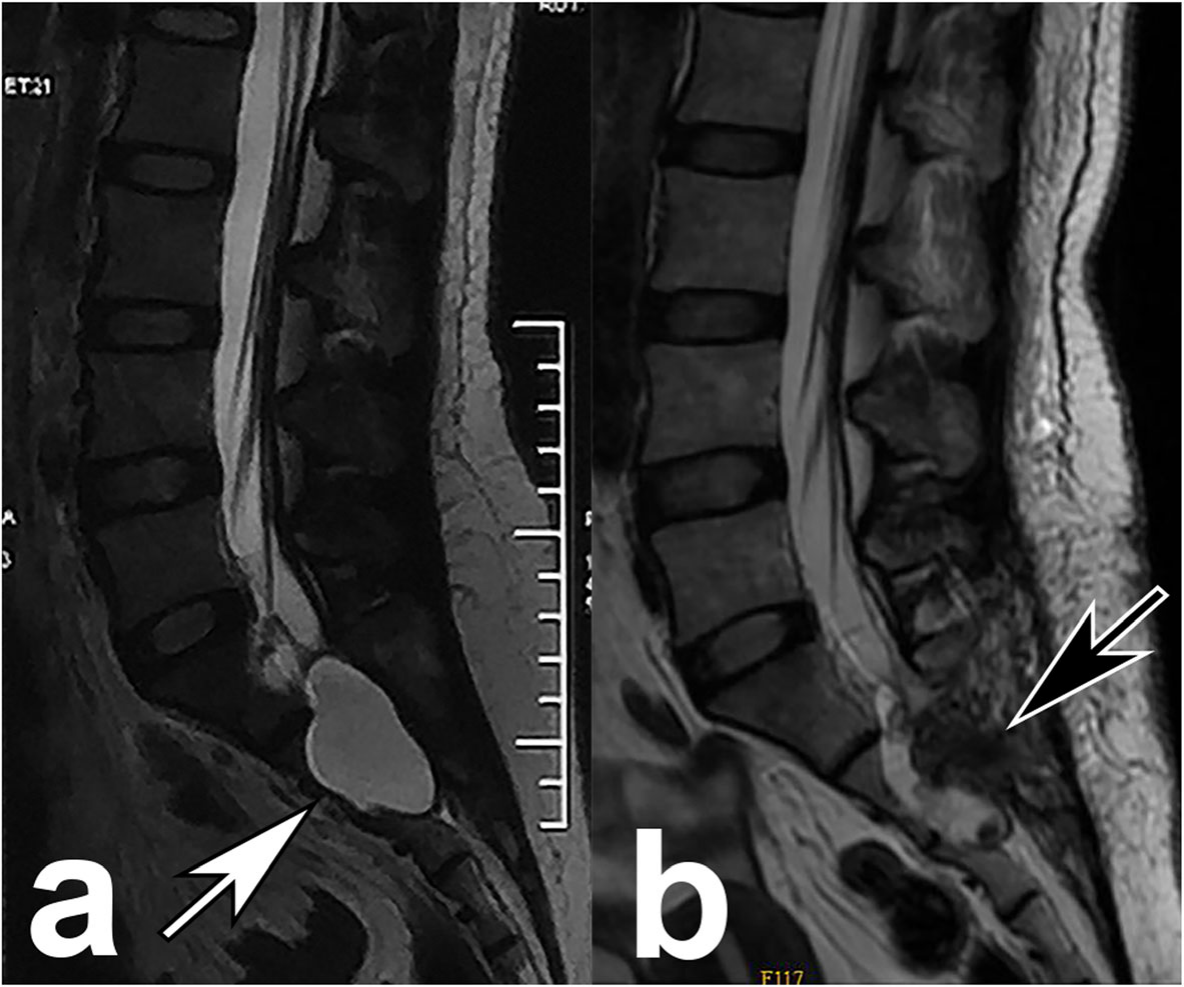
Magnetic resonance images before and after the operation: (a) preoperative image shows a sacral meningeal cyst at S1–3 level; (b) the image taken 2 days after surgery shows the obliteration of the cyst and the muscle graft (black arrow)
